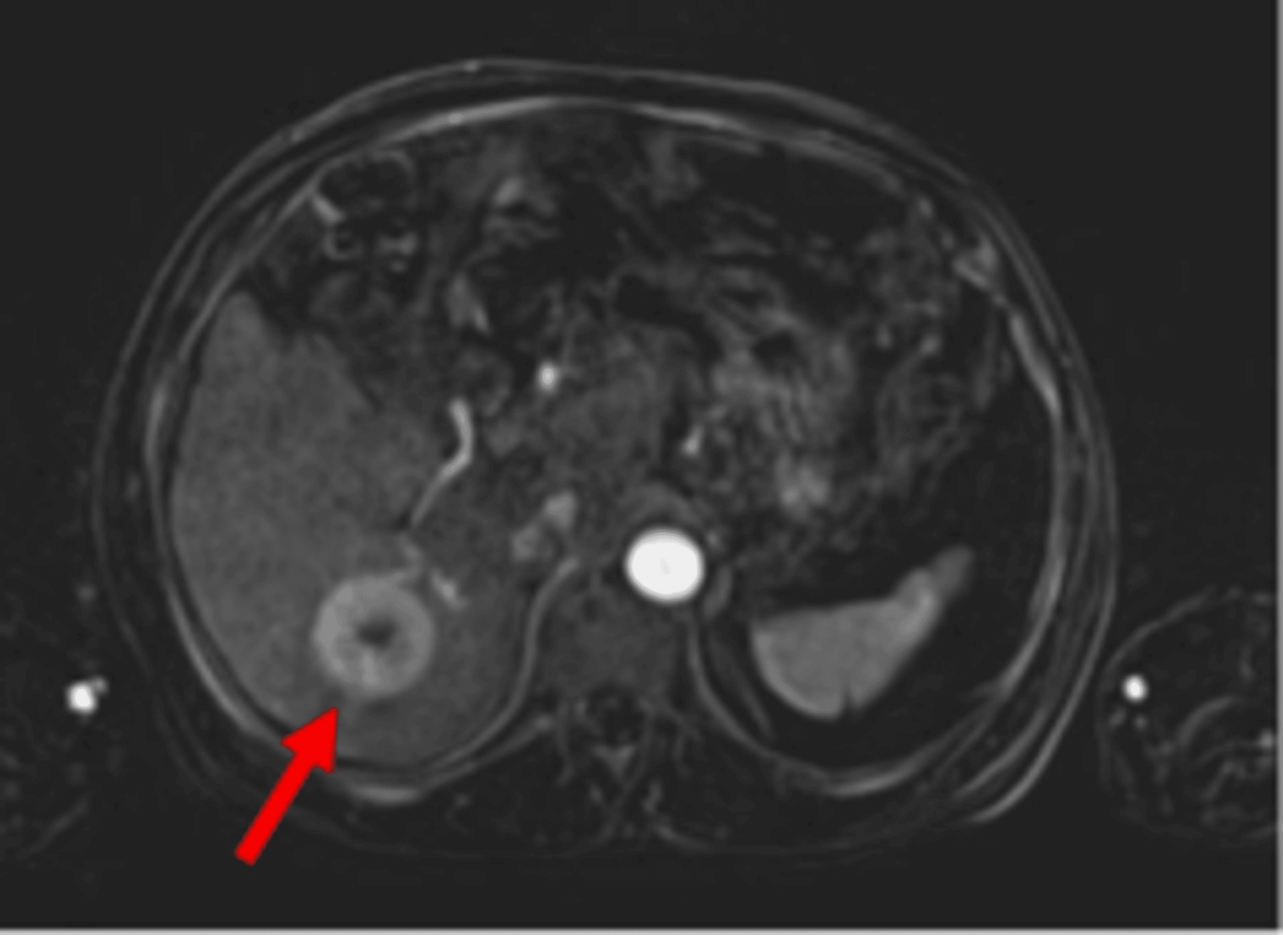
Axial MRI of the liver showing a 4.1 x 3.9 cm arterial enhancing lesion with delayed washout in the right hepatic lobe (red arrow), consistent with hepatocellular carcinoma. The presence of HCC influenced the decision-making regarding TIPS candidacy, given its association with poor prognosis and increased procedural risk. HCC: hepatocellular carcinoma; TIPS: transjugular intrahepatic portosystemic shunt
